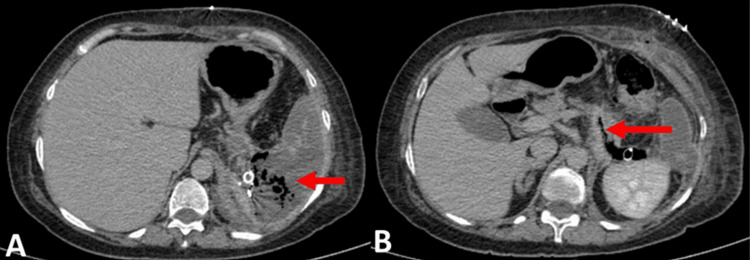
Contrast-enhanced computed tomography (coronal section). A. Spleen diffusely hypodense and non-enhancing. B. Few air foci through the parenchyma communicating with the collection.
